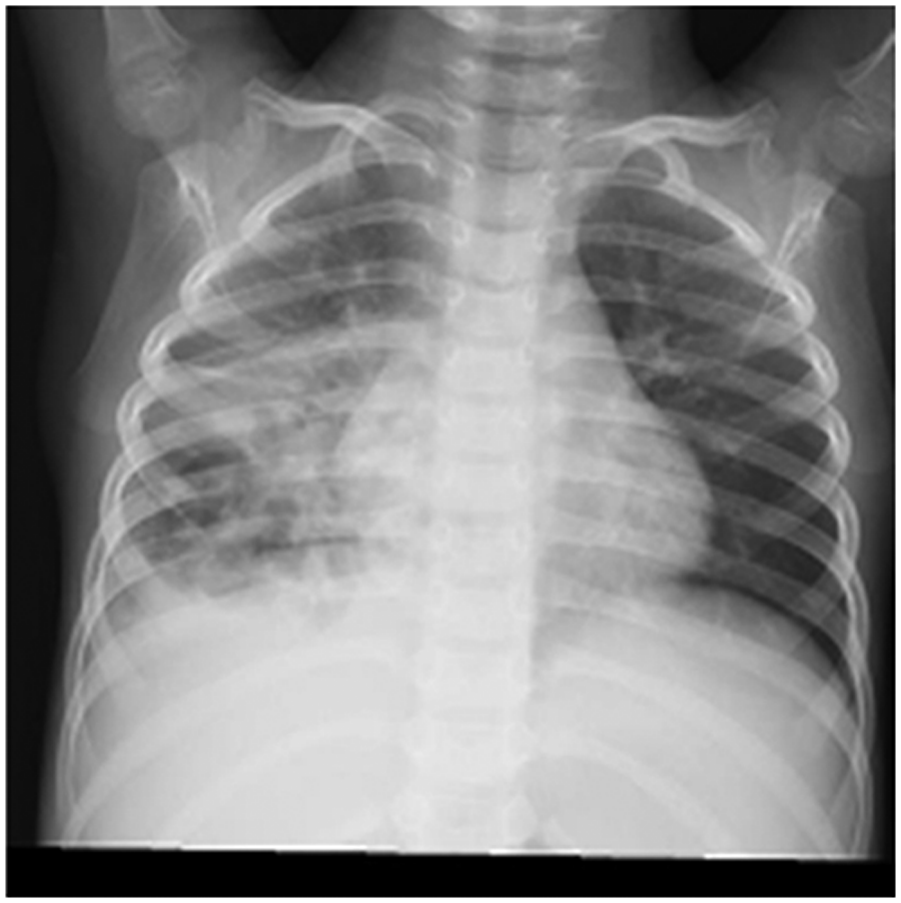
Chest x-ray findings on 21st of onset.
